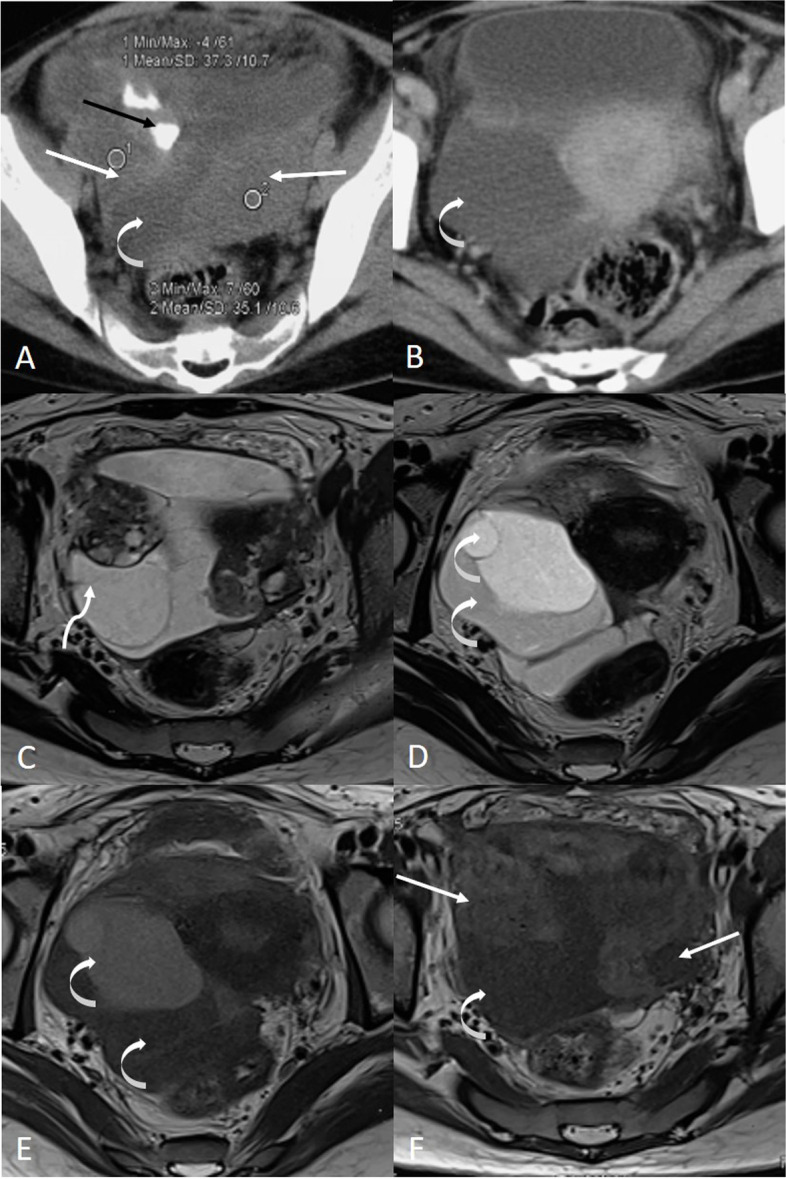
Axial MRI images of the pelvis before treatment showing the uterus in the center with a multiloculated cystic lesion being hypoattenuating on the CT scan (A, B), variably hyperintense on T2-weighted MRI (C, D), and variably hyperintense to hypointense on T1-weighted (E, F) images. The lesion also shows calcification on the CT scan (black straight arrow in A) and variable intensity pattern (curved arrows) suggesting that there are cystic components of various compositions. The lesion also shows a partial septa sign (twisted arrow in C), and this along with close circumferential relation with ovaries (straight white arrows in all sections) clearly indicated towards a possible tubo-ovarian origin of the mass. The bulk of both ovaries is however visualized normally (straight white arrows)
